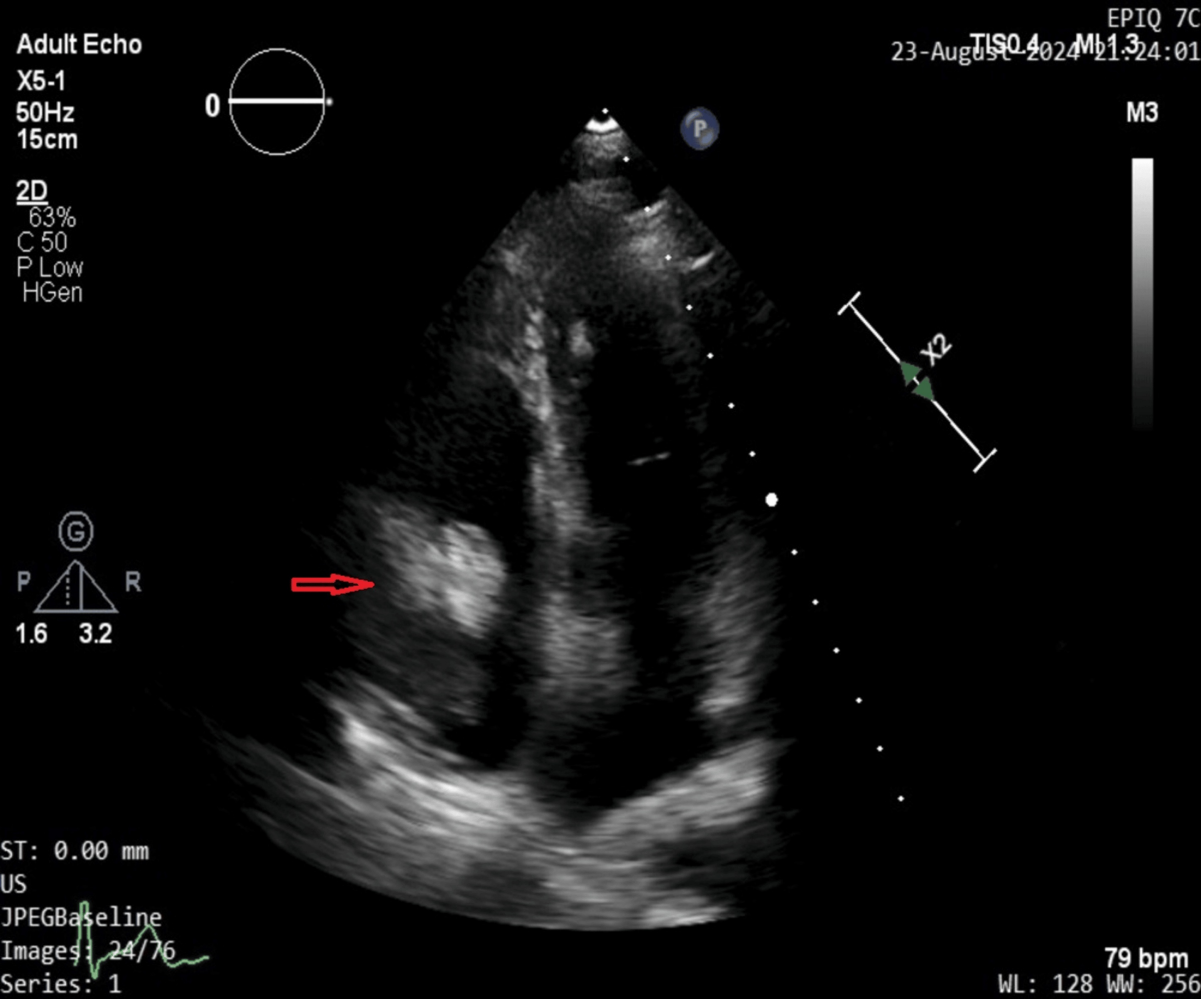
Transthoracic echocardiography at the end of four weeks suggesting persistence of the cardiac mass (red arrow) measuring 6 x 6 mm in the right atrium.
